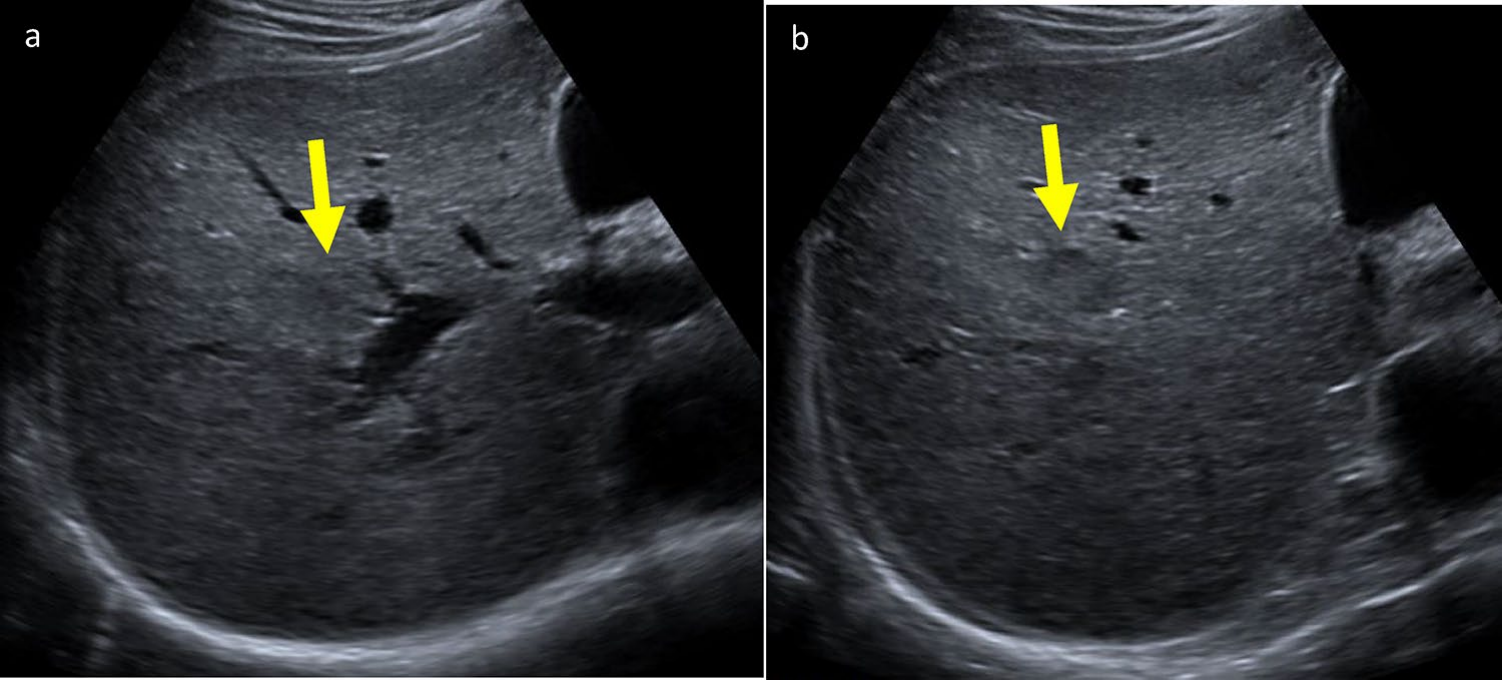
Cine sweeps clarify questionable finding on static grayscale images in a 63-year-old male with advanced fibrosis undergoing HCC surveillance. a Transverse grayscale ultrasound image shows a possible hypoechoic area (arrow) adjacent to right portal vein. b Select transverse grayscale image from a transverse right lobe cine sweep confirms a 2 cm solid hypoechoic nodule (arrow) that was categorized as LR-M on subsequent CEUS
